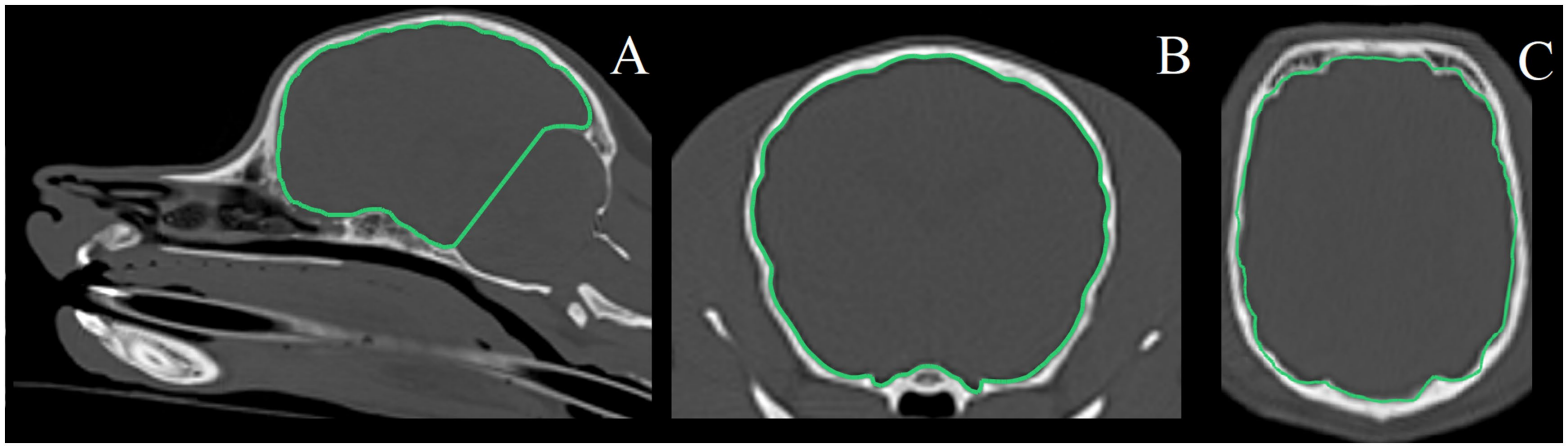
Example of segmentation of volume of interest (rostral and middle cranial fossa volume) on CT images. (A) Sagittal plane, (B) transverse plane, (C) dorsal plane.
